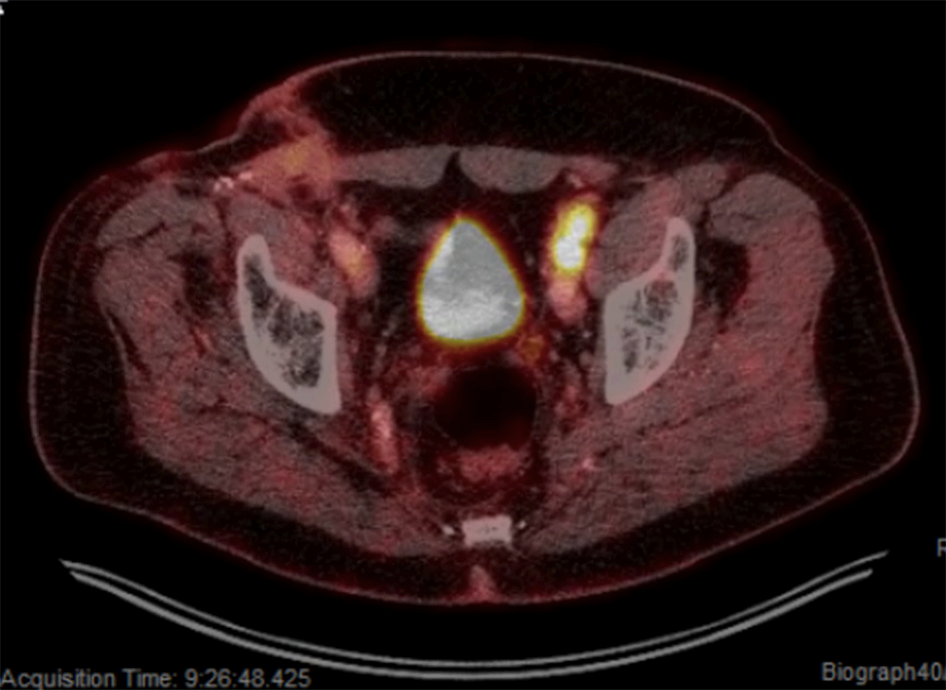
Isolated left pelvic nodal progression on pembrolizumab. PET-scan showing SUV avid progression involving the left inguinal and left external iliac regions.
